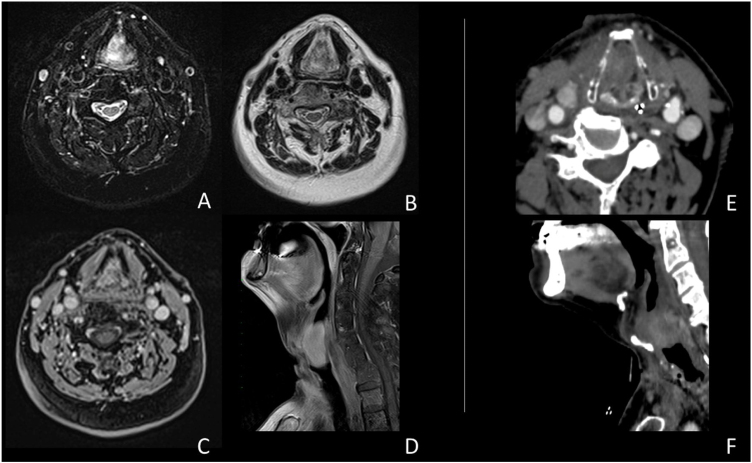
(A‒F) MRI study (A‒D); CT study (E‒F). The images document an expansive glottic lesion characterized by homogeneous enhancement after contrast injection in CT and MRI studies. In addition, obliteration of the glottic space is evident, with anterior dislocation of the thyroid cartilage shield without visible involvement of the paraglottic area and the cartilaginous structures.
